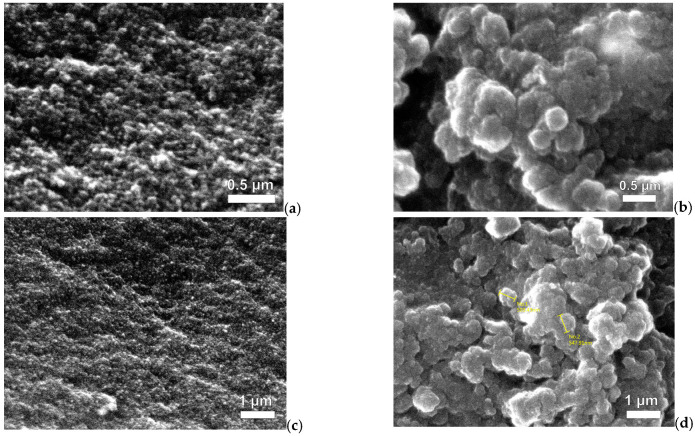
SEM mages of Fe_3_O_4_-monolithic resorcinol-formaldehyde xerogels prepared by (a,c) direct (MX1) and (b,d) indirect (MC4) ultrasonication with magnifications.
